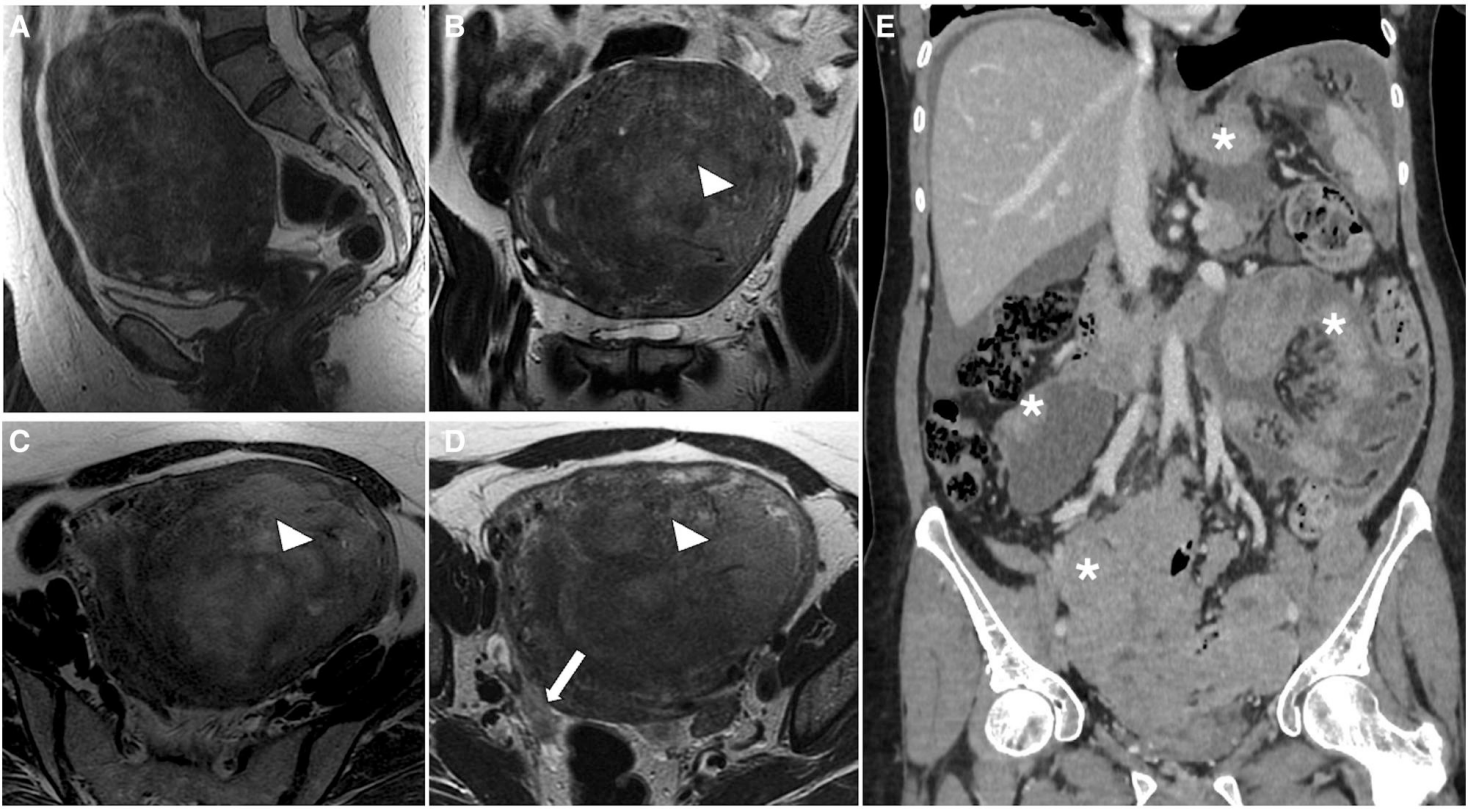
High-grade endometrial stromal sarcoma. Sagittal (A) and axial T2-WI (B-D) show the typical appearance of a high-grade ESS with hypointense bands of preserved myometrium within areas of myometrial involvement (arrowheads). The tumour extends through the right fallopian tube (arrow) into the pelvic peritoneum (D). The aggressive nature of the tumour is demonstrated by the diffuse peritoneal involvement seen on CT (asterisks in E). Abbreviations: ESS = endometrial stromal sarcoma; WI = weighted imaging.
